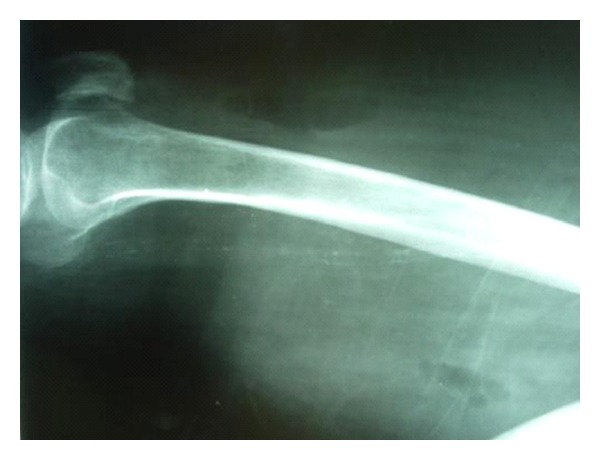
Plain lateral radiograph shows the right femur. Subcutaneous emphysema is observed as gas in the fascial planes between muscles and subcutaneous tissues.
